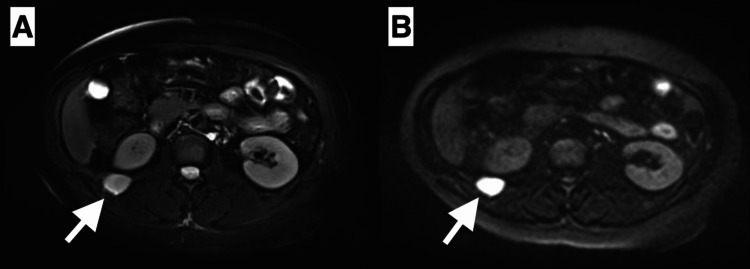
A right paraspinal intramuscular nodule (arrow) that has hyperintensity on fat-sat T2-weighted image (A), and has diffusion restriction on the diffusion-weighted image (B) was revealed.
